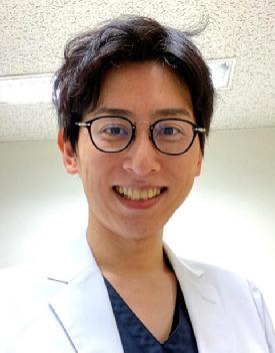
Dr Hiroto Yagasaki is a cardiologist at Gifu Prefectural General Medical Center, Japan, and a visiting researcher at Indiana University School of Medicine, USA. He specializes in echocardiography and cardiac magnetic resonance imaging, focusing on structural heart disease interventions. At his institution in Japan, he leads procedures including transcatheter aortic valve implantation, edge-to-edge valve repair, left atrial appendage closure, and patent foramen ovale closure. He holds board certification in internal medicine, cardiology, echocardiography, and other cardiovascular subspecialties in Japan.
